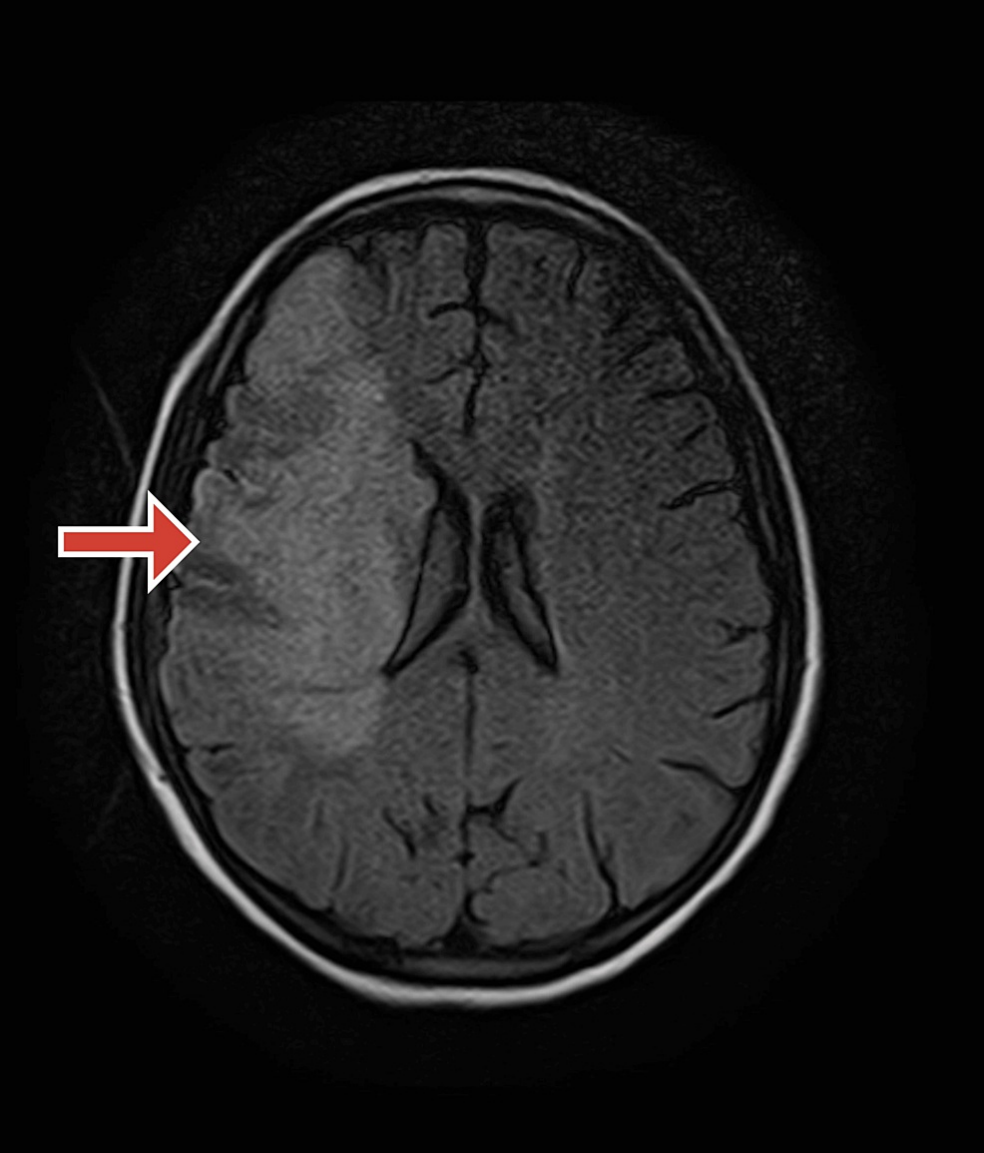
T1 MRI image with an arrow pointing to hemorrhagic infarct in the territory of the middle cerebral artery (MCA)
